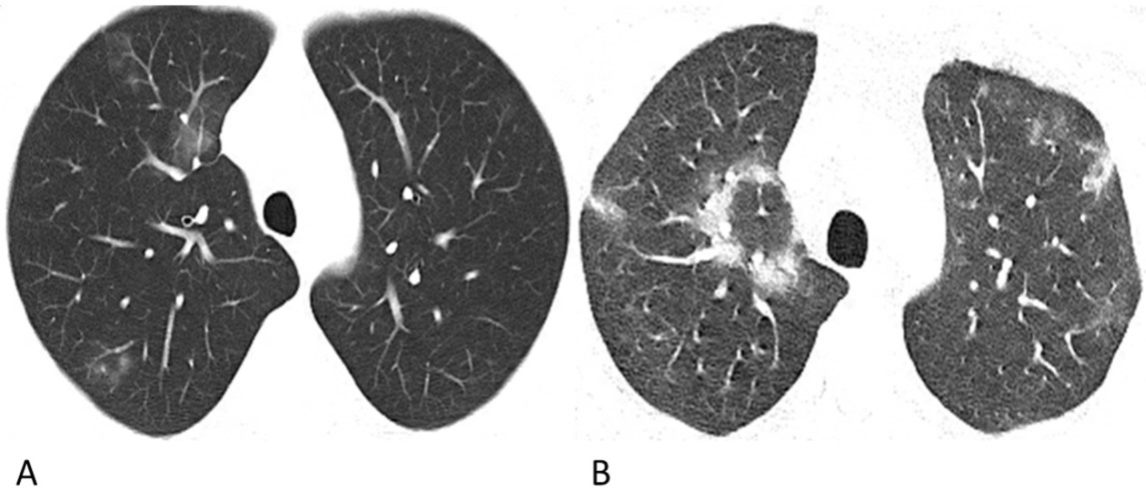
Representative chest CT images of COVID-19 pneumonia with halo sign (A) and reversed-halo sign (B). (A) A 28-year-old man who worked in Wuhan had a fever and cough on admission. A Patchy GGO and two mixed GGO nodules are shown in the right upper lobe, one of which with halo sign. (B) A 32-year-old man who lived in Wuhan developed fever and cough. Reversed-halo sign is shown in the right upper lobe.
